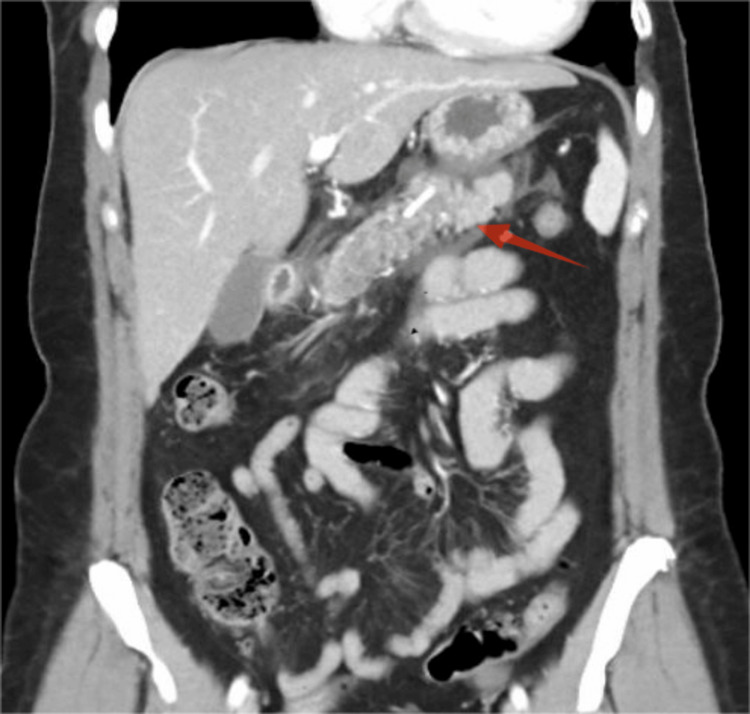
Contrast-enhanced CT of the abdomen and pelvis revealing diffuse acute pancreatitis without necrosis CT: computed tomography
